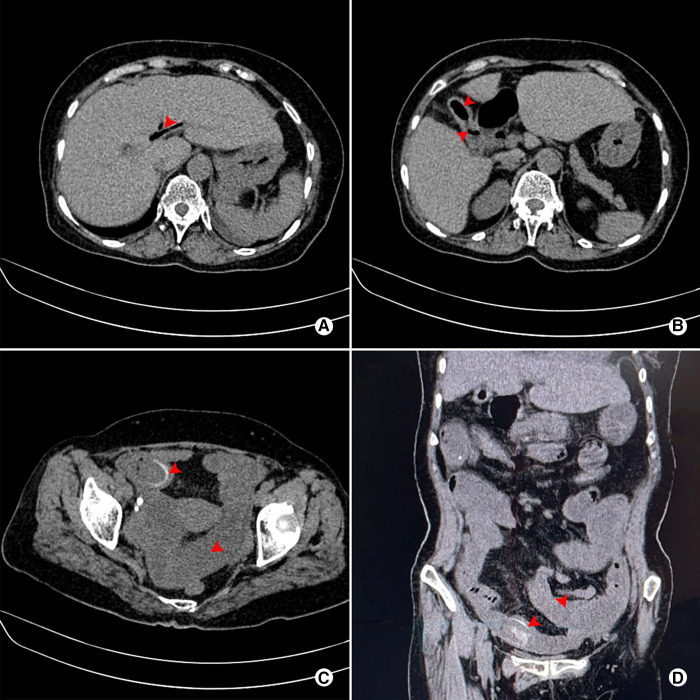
Abdominal plain CT scan of a 71-year-old female patient who was preoperatively diagnosed with CDF combined with GI. (A) Pneumobilia (red arrow); (B) Pneumo-gallbladder and ill-defined borderline between gallbladder and digestive tract (red arrow); (C) Rim-calcified gallstone impacted in the small bowel, combined with dilatation of proximal small bowel (red arrow); (D) Coronal view of impacted rim-calcified gallstone and dilatation of proximal small bowel (red arrow).
